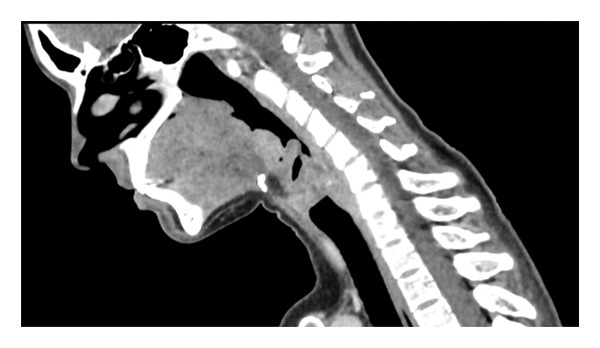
Sagittal cut of cervical CT scan with a thickening of the laryngeal mucous membranes.
